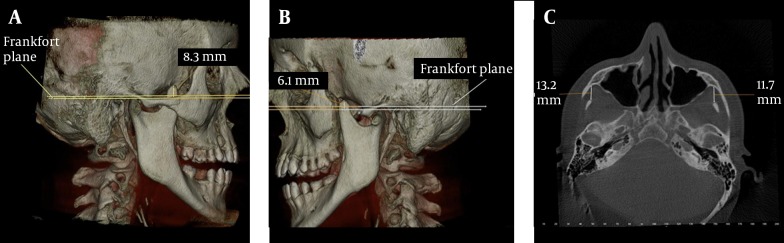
Case 3; A, 3D view of the elongated right coronoid process; B, 3D view of the elongated left coronoid process; C, The distance between the coronoid process and the inner face of the frontal part of the zygomatic bone on axial CBCT scan
